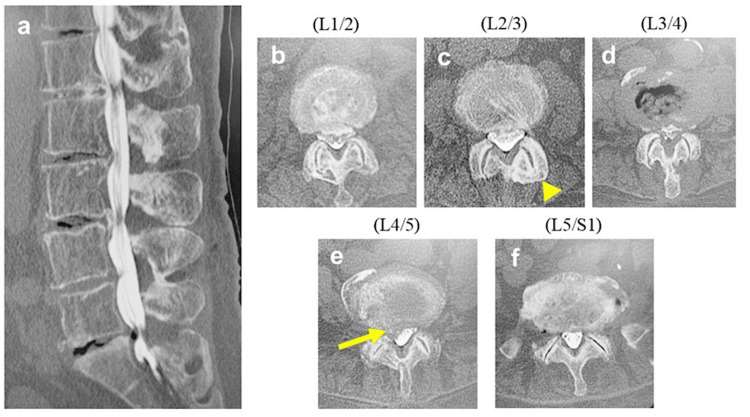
Preoperative CT myelography (a) Sagittal image of lumbar spine. (b) Axial image of L1/2. Lumbar spinal canal stenosis was shown. (c) Axial image of L2/3. The L2/3 facet with partial union was shown (yellow arrowhead). (d) Axial image of L3/4. Lumbar spinal canal stenosis was shown. (e) Axial image of L4/5. L4/5 calcificated herniation was shown (yellow arrow). (f) Axial image of L5/S1.
